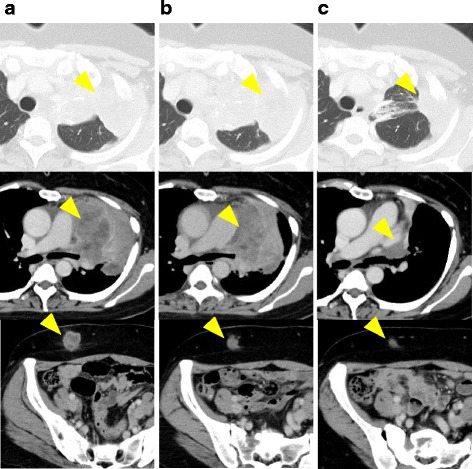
Computed tomography images demonstrate a decrease in the size of lung nodules, lung hilar lymph node metastases, and skin metastases after four and twelve cycles of nivolumab. a Before initiating nivolumab therapy. b After four cycles of nivolumab. c After twelve cycles of nivolumab
